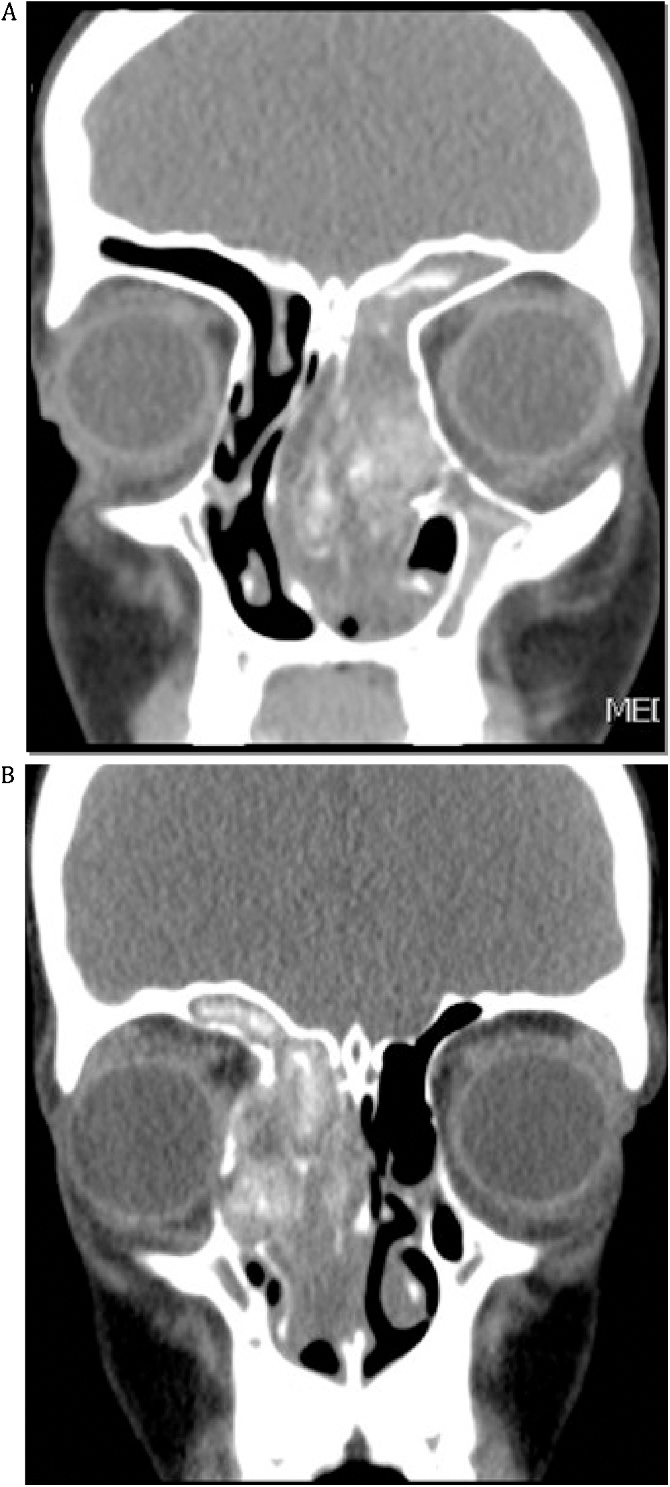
(A) Coronal CT image shows heterogeneous opacity filling the left maxillary and frontoethmoidal sinuses with bone expansion and pushing the septum to the right side. (B) Coronal CT image post-surgery shows disease recurrence in the right sinuses and clean left side.
